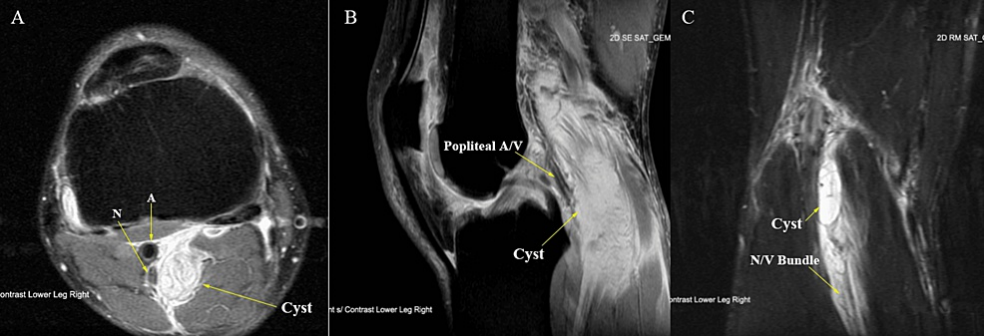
Magnetic resonance imaging in the axial (A), sagittal (B), and coronal (C) planes showing the close proximity and compression of the popliteal cyst against the posterior neurovascular structures. A: Artery; N: Nerve; A/V: Artery and Vein; N/V: Neurovascular; Cyst: Popliteal Cyst of Interest.
